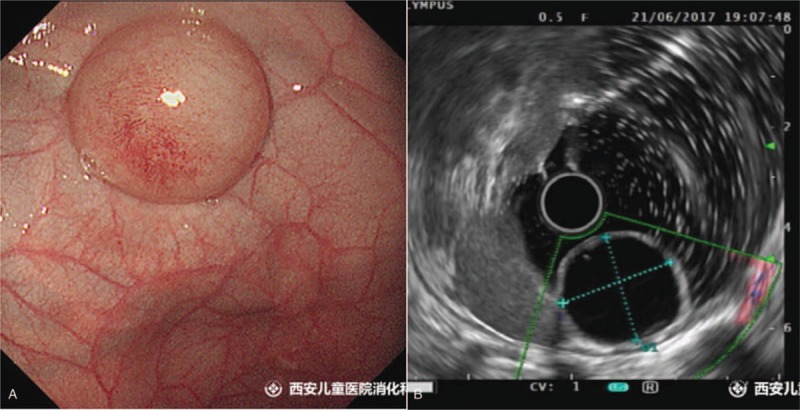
A, Upper endoscopy showed bulging lesions. B, Endoscopic ultrasonography showed that the lesion originated from the submucosal layer.
